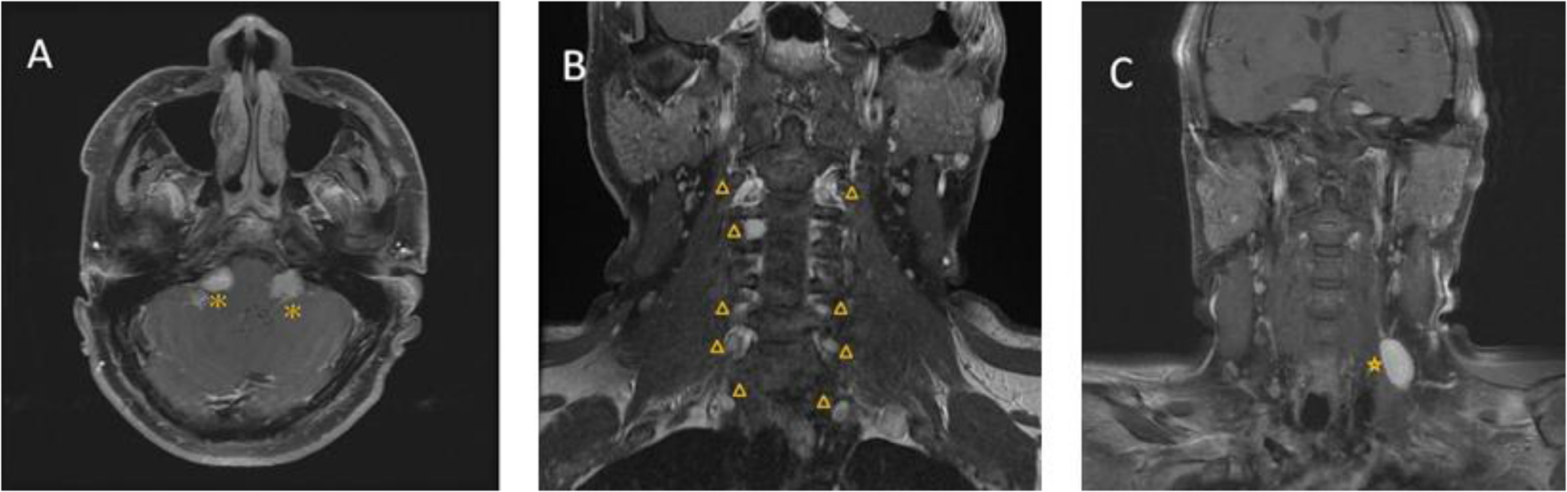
T1-weighted MRI with gadolinium enhancement (a: axial view, b, c: coronal views) showed bilateral vestibular schwannomas (*), multiple tumors over the bilateral cervical neuroforamens (△) and left posterior cervical neck (★)
